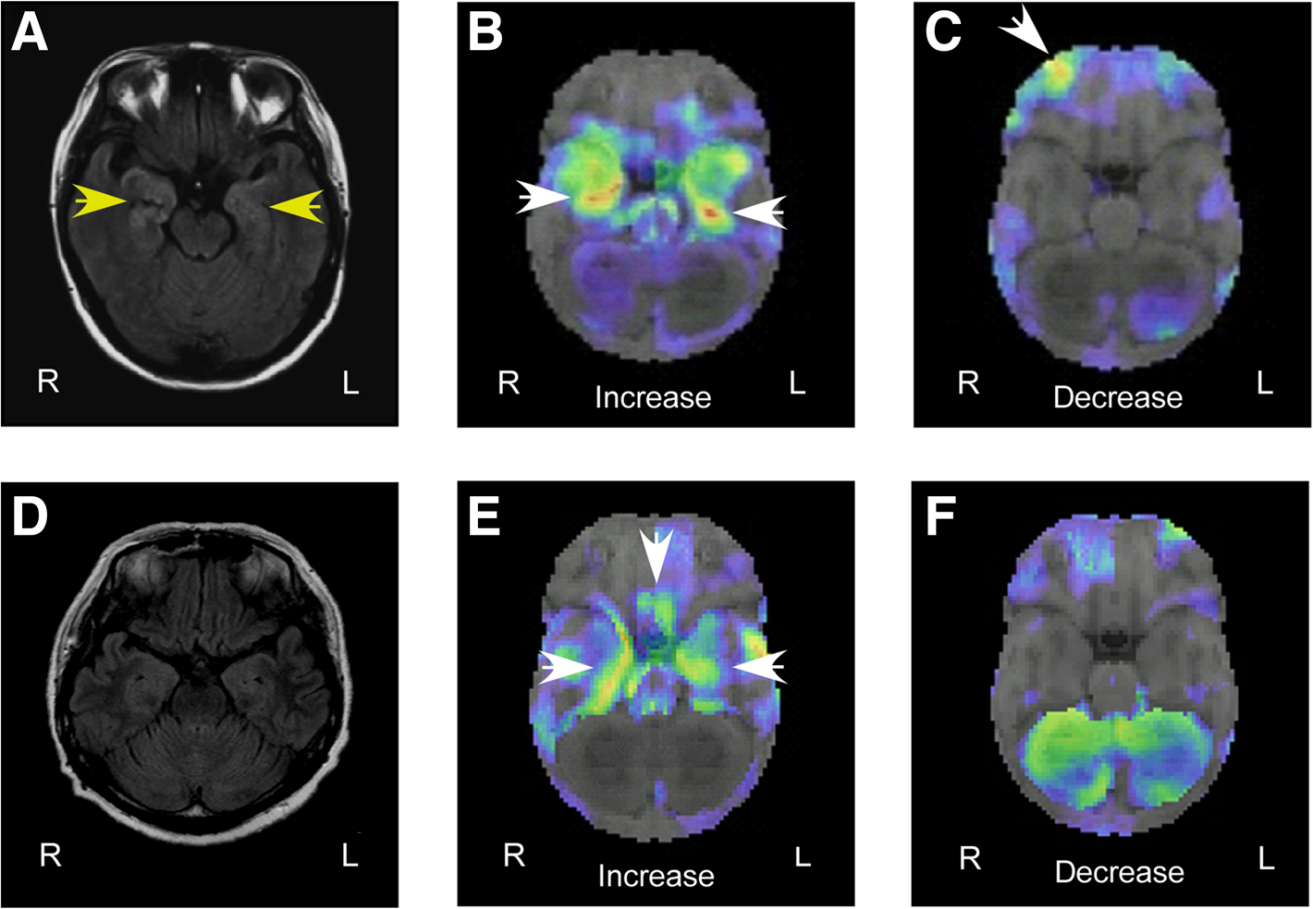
Imaging data. Case 1 (a–c). High-intensity signal in bilateral medial temporal lobes as shown by magnetic resonance imaging, axial fluid-attenuated inversion recovery image (a). Increased blood flow in bilateral medial temporal lobes (b), with decreases in the right orbitofrontal cortex (c), as assessed by ^123^I-IMP brain perfusion single-photon emission computed tomography with three-dimensional stereotactic surface projection analysis. Case 2 (d–f). No specific findings on the magnetic resonance imaging, axial fluid-attenuated inversion recovery image (d). Increased blood flow in bilateral medial temporal lobes and the posterior orbitofrontal cortex (e), with no decreases (f), as shown by ^123^I-IMP brain perfusion single-photon emission computed tomography with three-dimensional stereotactic surface projection analysis
